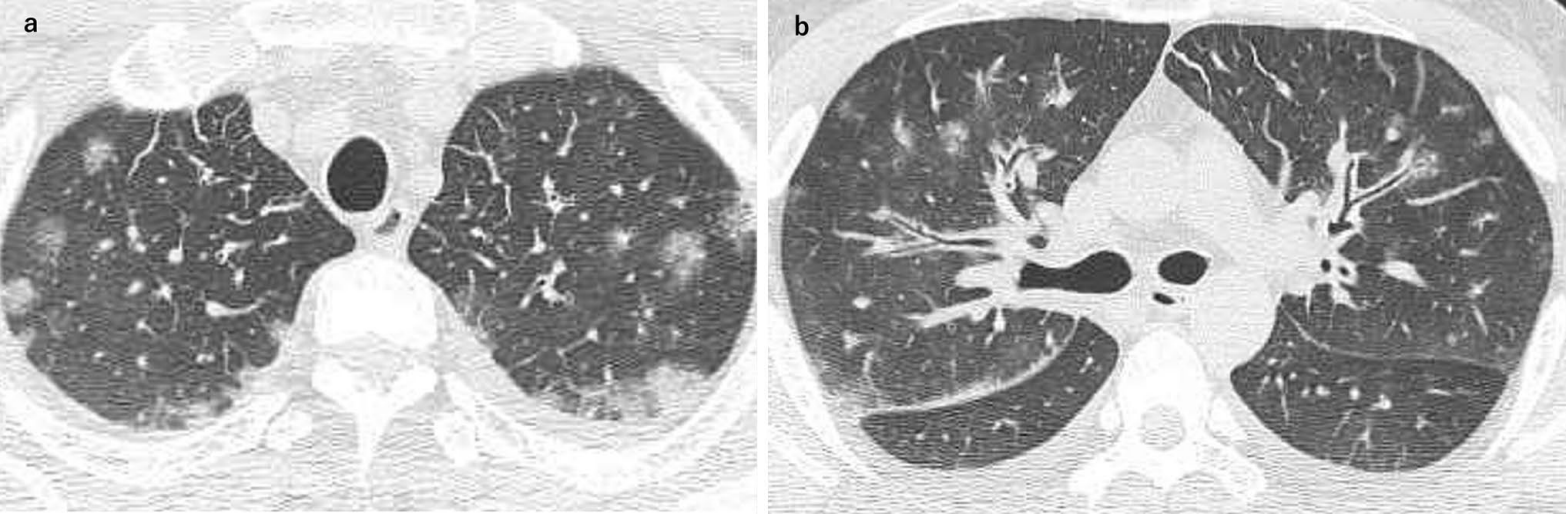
Decompression illness. A male fisherman in his 30 s surfaced after working at a depth of 35 m. At 30 min after he came to the surface, he experienced numbness in his hands and feet, followed by respiratory symptoms. He was diagnosed with decompression illness. Chest CT revealed multiple peripheral ground-glass nodules in both lungs, along with interlobular septal thickening (a) and thickening of the bronchovascular bundles (b)
